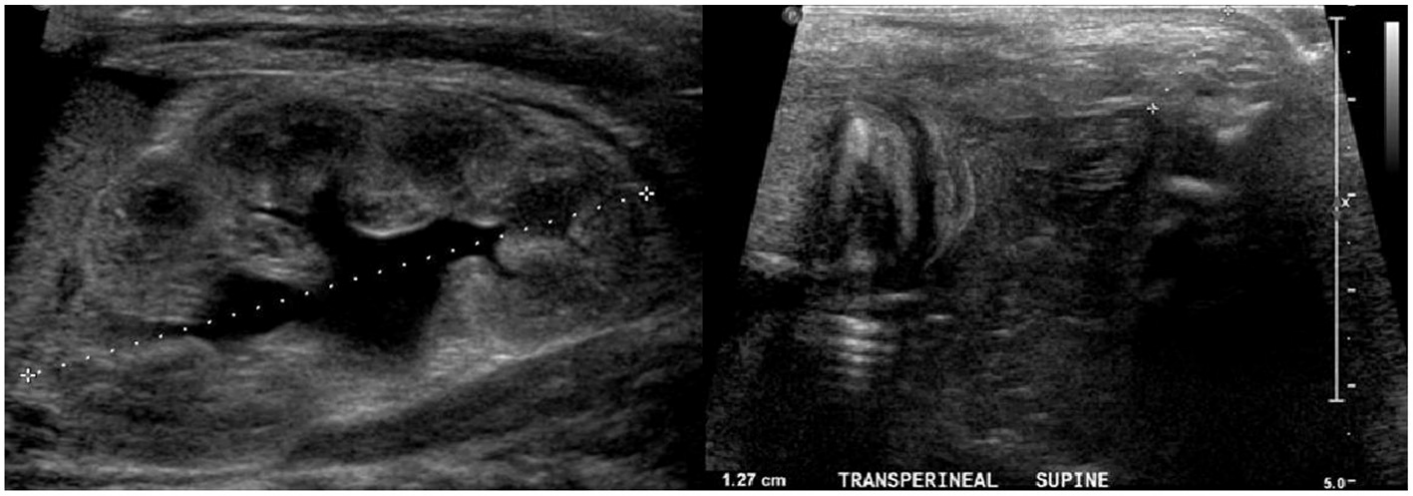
Ultrasound of the kidneys, ureter, bladder, and perineum demonstrated a dilated left pelvicalyceal system. There was a distal rectal pouch to the perineum that measured 12 mm.
